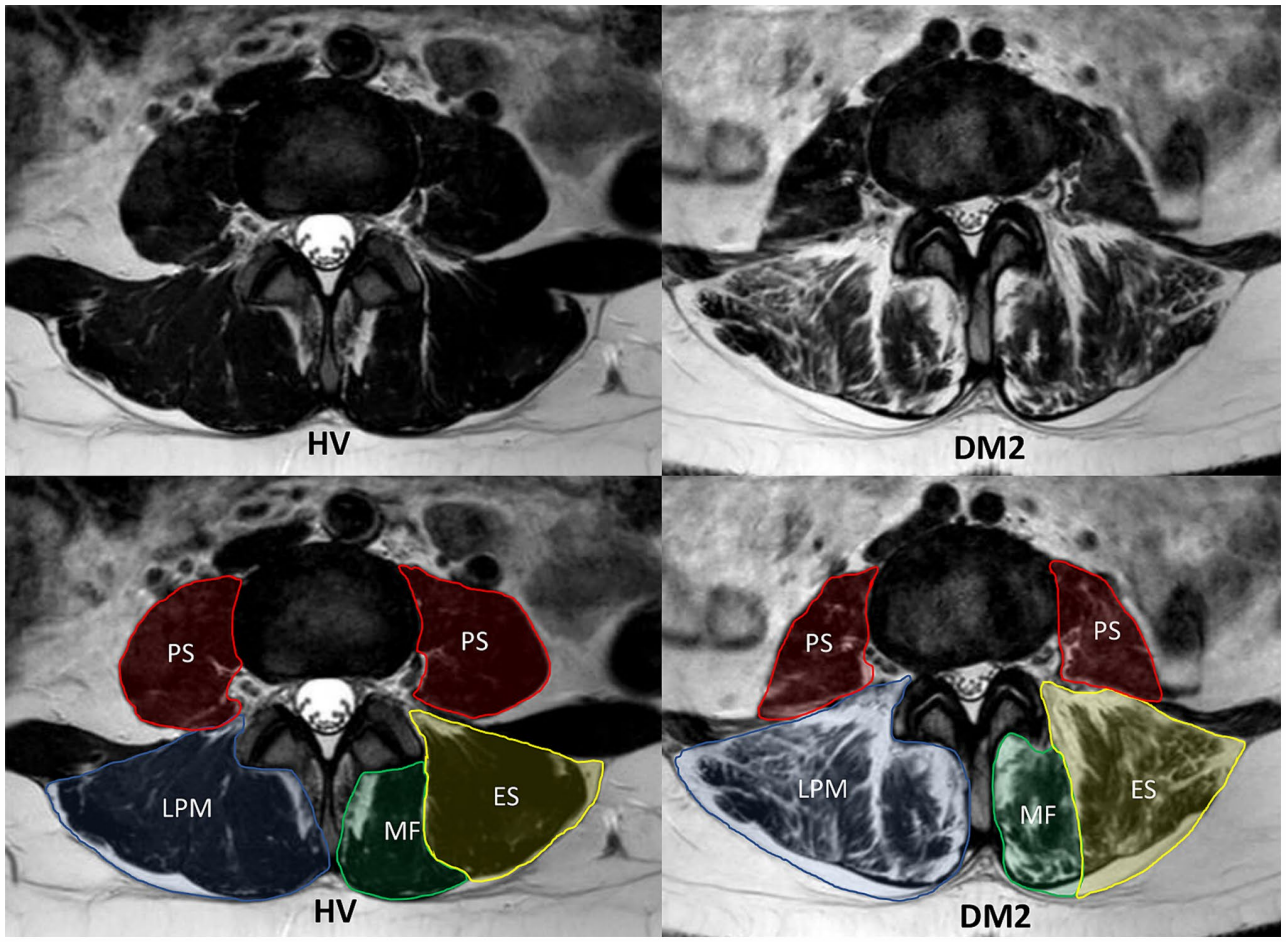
MRI T2-weighted sequence of the lumbar paraspinal muscles (LPM) and psoas muscles (PS) from a matched pair (patient with myotonic dystrophy type 2 (DM2) on the right and healthy volunteer (HV) on the left). The figures show MRI images in the axial plane at the same level of the intervertebral disk at L3/L4. In the lower figures, the individual segmented muscles are delineated. The LPM include the erector spinae muscle (ES) and the multifidus muscle (MF).
